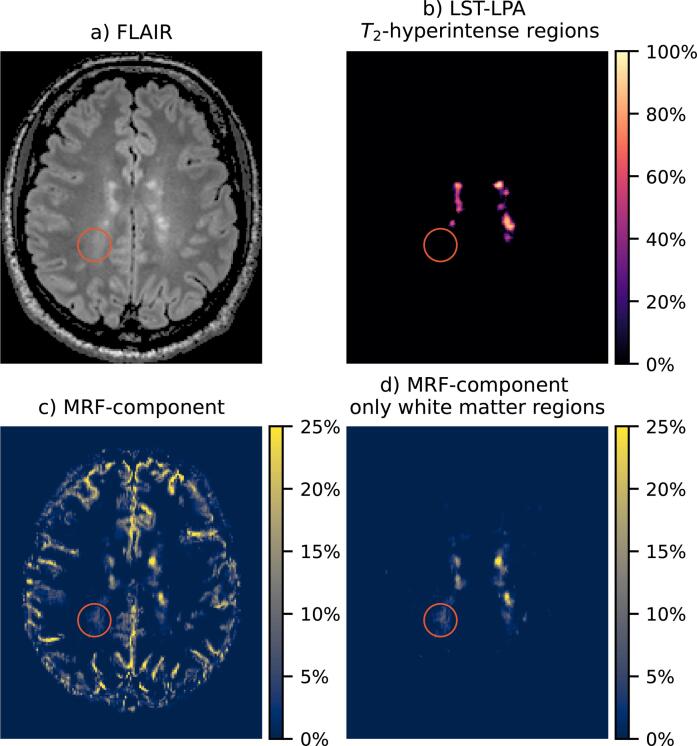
Results from a single slice of an MS patient. A region with DAWM is indicated by the red circle. a) FLAIR scan, b) estimated lesion probability map from the FLAIR scan, c) MRF-component with long before masking and d) after masking non-white matter regions. (For interpretation of the references to color in this figure legend, the reader is referred to the web version of this article.)
